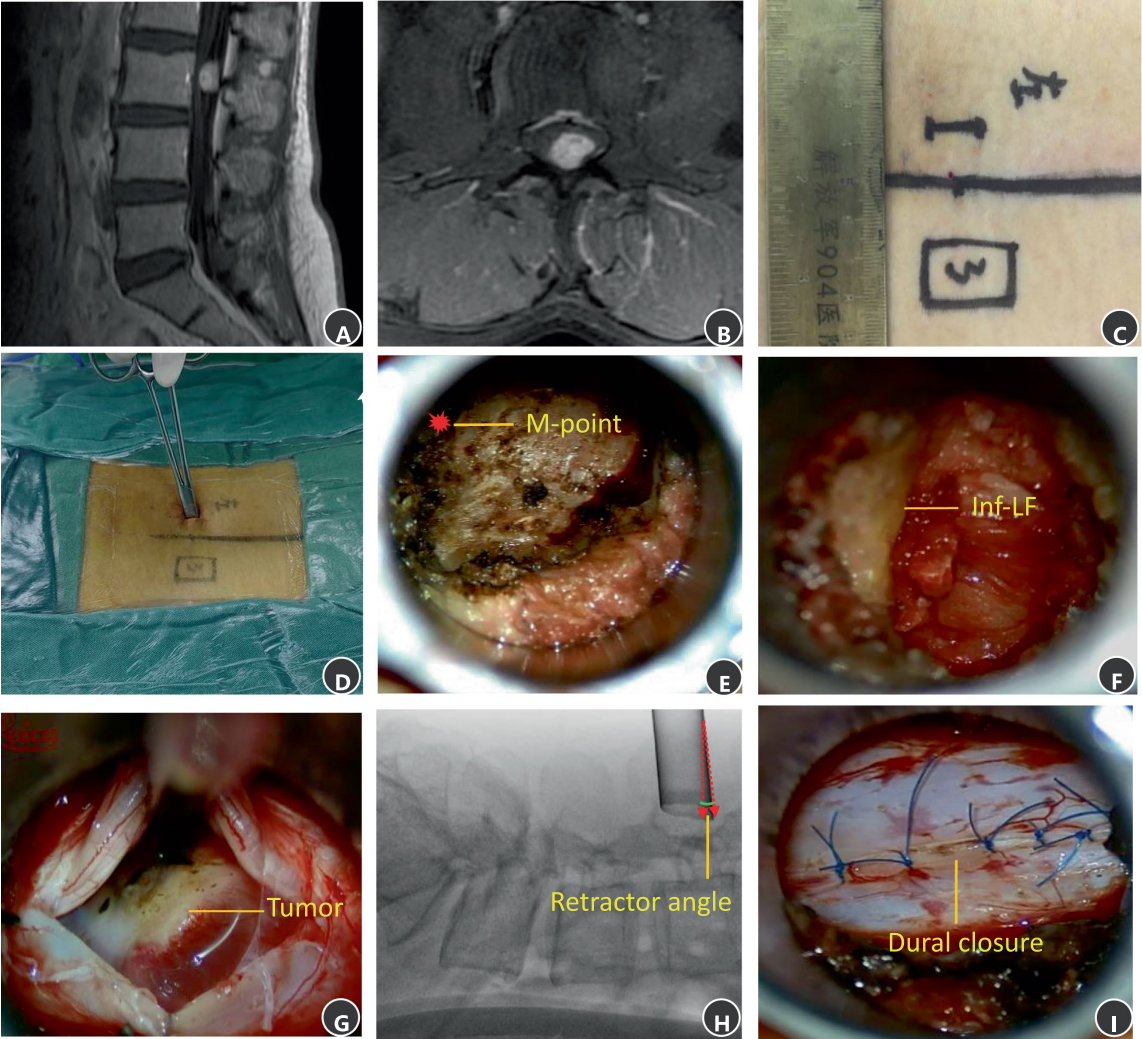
MRI and intraoperative surgical photos of schwannoma at the L3 level. (A) A sagittal T1 contrast image. (B) An axial T1 contrast image. (C) Surgical incision. (D) Palpating the M-point. (E) The M-point lies at the cephalad aspect of the operative field. (F) The inferior boundary of previous segment ligamentum flavum at the cephalad aspect of the operative field. (G) The main tumor mass is exposed and visualized at the center of the surgical corridor. (H) An intraoperative X-ray image showing retractor angle after exposed the main tumor mass. (I) Interrupted suture of the dura.
